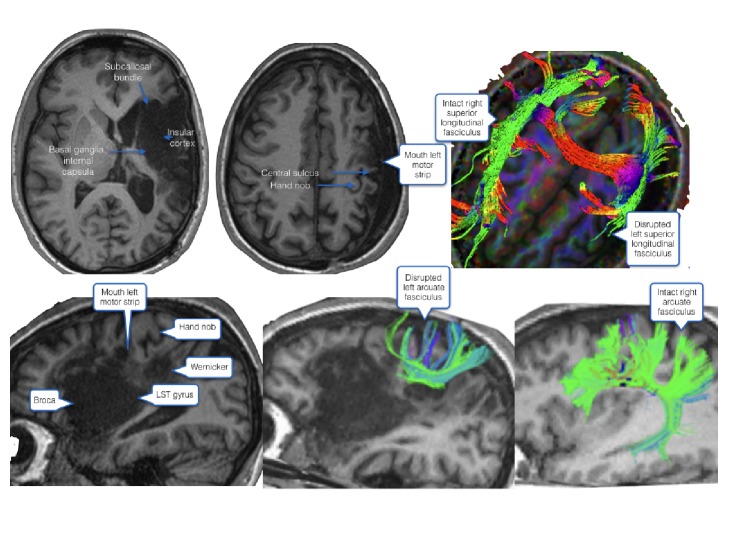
Poststroke brain lesions are shown in 3T MRI.
